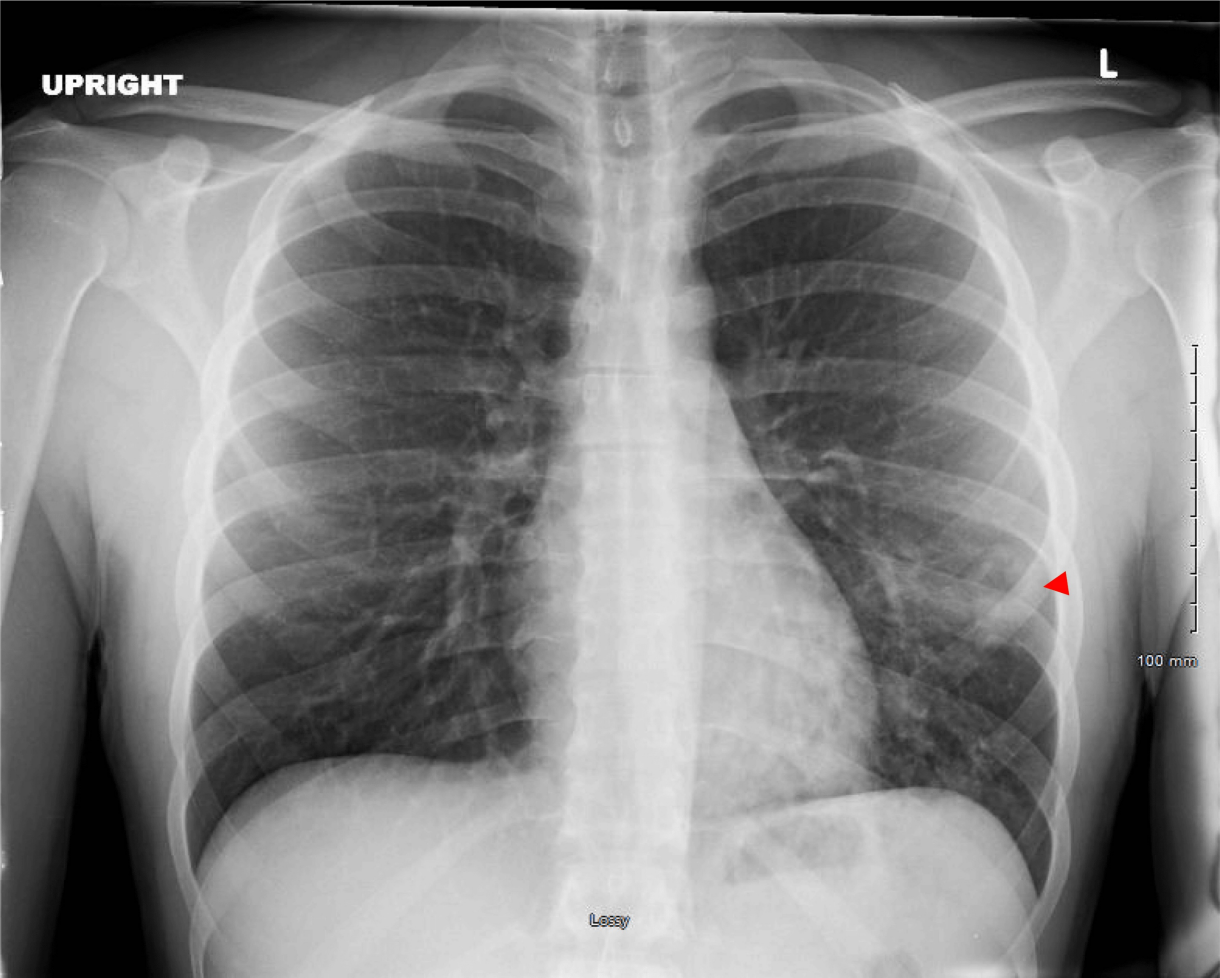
Portable One-View Chest X-ray (Anteroposterior View) Left lower lobe pneumonia with suspected cavitary lesion (red arrowhead) in the peripheral mid-to-lower left lung. Contrast-enhanced CT imaging of the chest was recommended for further evaluation.
